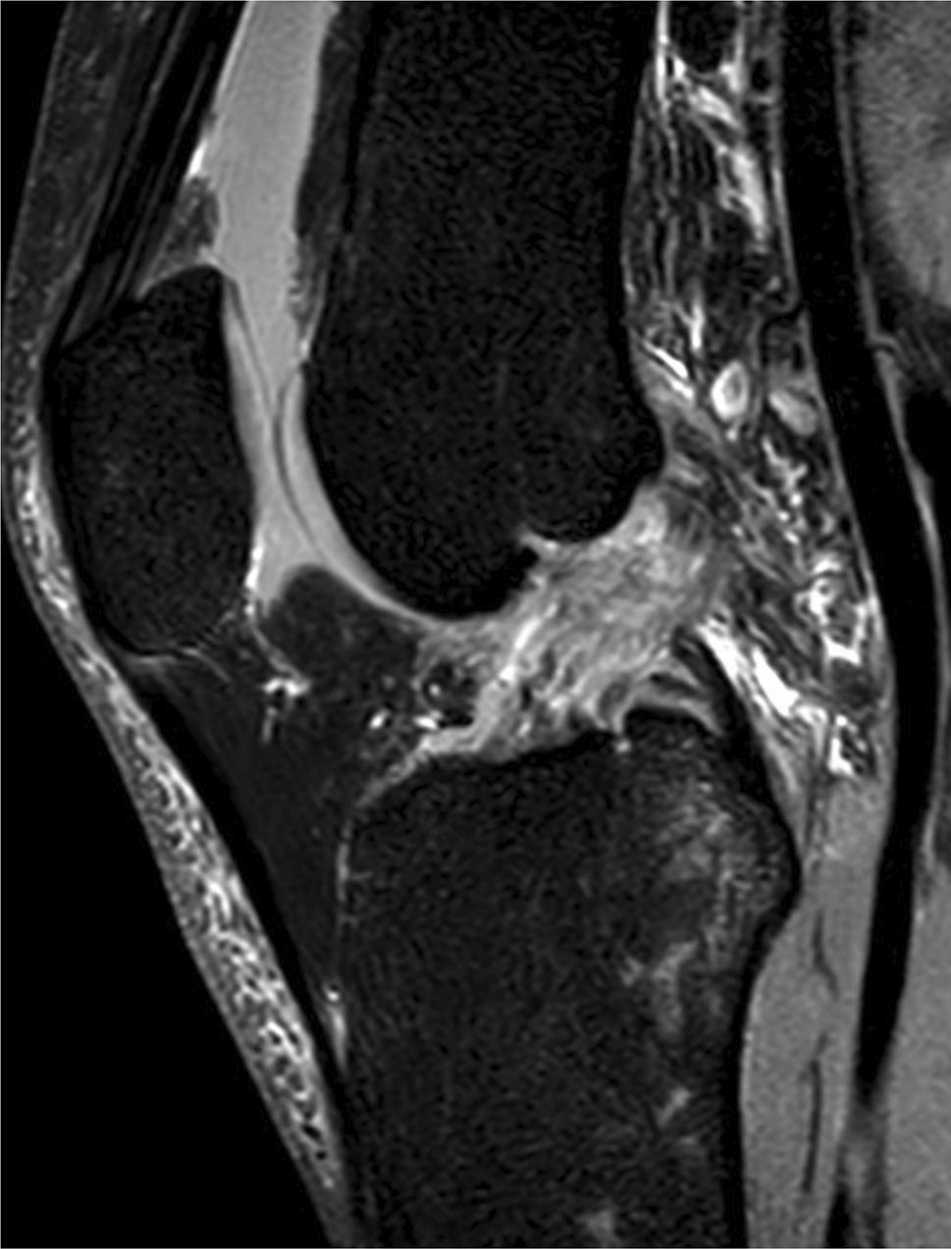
MRI scan of a left Knee in saggital plane with a ACL rupture
